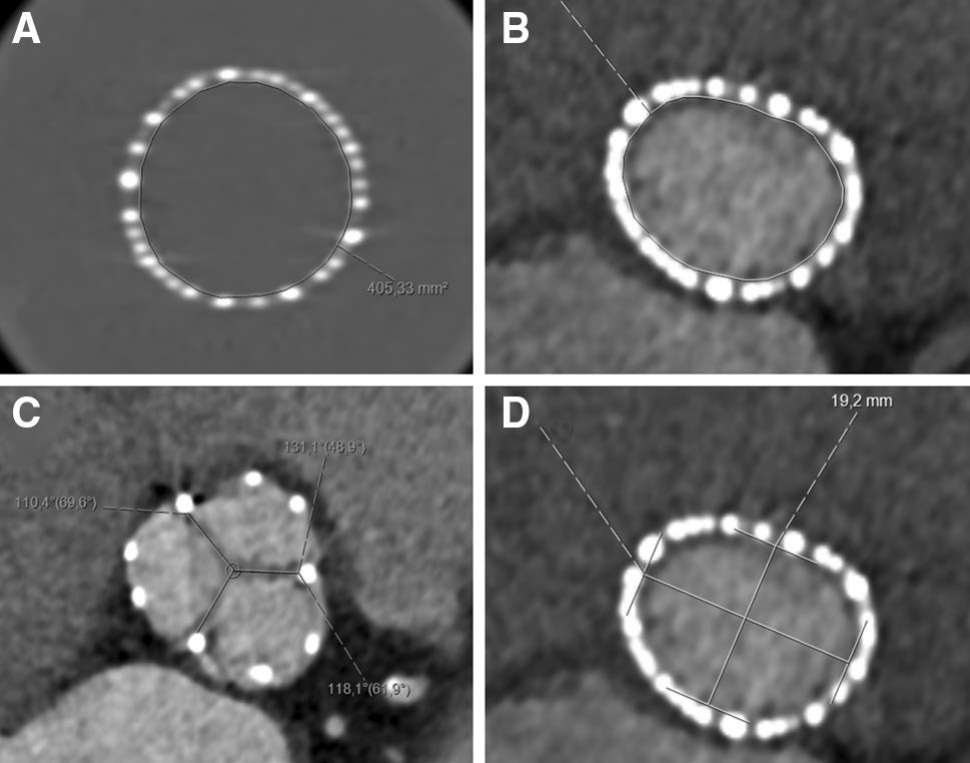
Cardiac computed tomography analyses. a Measurement of the in vitro cross-sectional area in a Perceval bioprosthetic valve. b Measurement of in vivo cross-sectional area in an implanted bioprosthesis. The degree of overall expansion of the implanted prosthesis was calculated as the ratio between the in vivo cross-sectional area (CSA) and the in vitro CSA of the implanted valve according to the formula (in vivo CSA/in vitro CSA) × 100. c Regional expansion measured using the angle (°) formed by the border of each prosthetic leaflet and the center point of the valve. d The degree of eccentricity (%) was calculated as ([max diameter–min diameter]/max diameter) × 100
